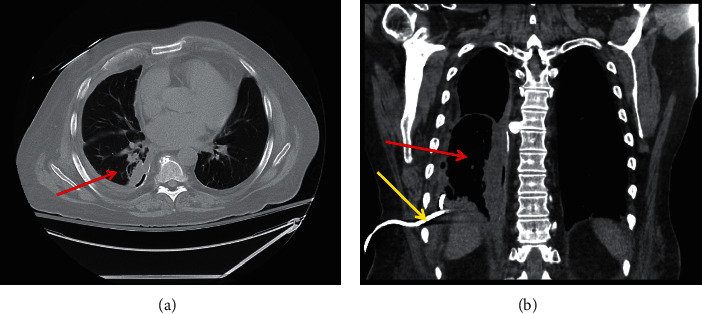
CT chest of the patient after chest tube placement in the (a) axial and (b) coronal views, suggesting progressive resolution. The red arrow denotes resolving pleural effusion. The yellow arrow denotes the chest tube.
